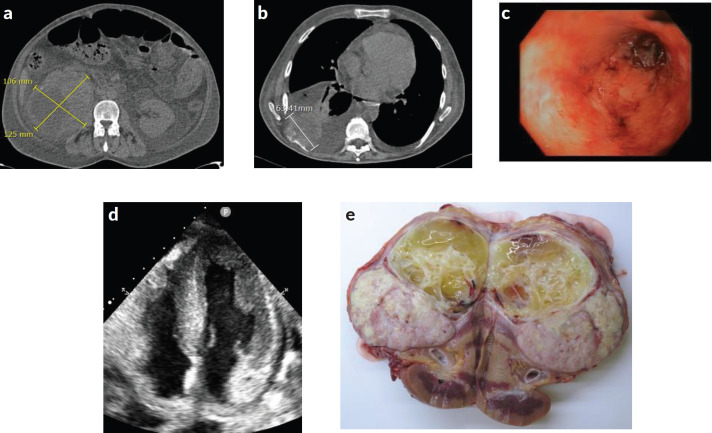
(a) and (b): Contrast-enhanced whole-body CT scan revealing stabilisation of the right renal mass, new bilateral pleural effusion and new pericardial effusion with an increase in the size of the lung metastasis (6 cm). (c): Lower gastrointestinal endoscopy demonstrating a severe pancolitis. (d): Echocardiography showing cardiac amyloidosis pattern. Increase in the thickness of the interventricular septum and walls of the ventricles. (e): Surgically removed piece from nephrectomy showing renal tumour.
